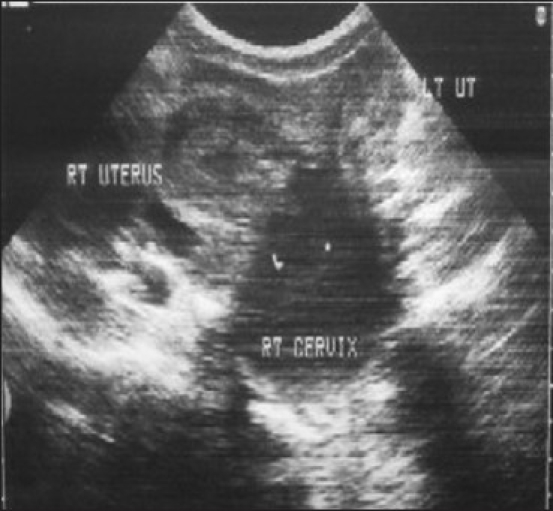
Transabdominal ultrasound showing two widely divergent uterine horns with no communication between them and a distended right cervix
